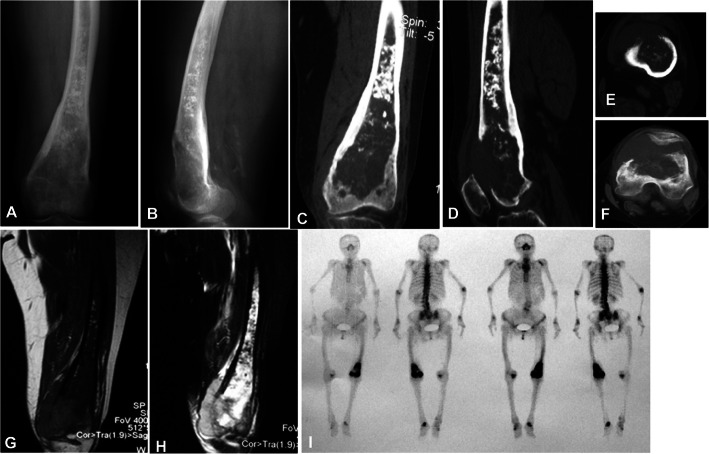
A 75-year-old woman had left leg pain for half a year and swelling of left knee for 5 months and was diagnosed to have dedifferentiated chondrosarcoma. a-f Plain radiograph (a and b) and computed tomography (c-f) displayed calcification within the medullary cavity, osteolytic destruction in the distal end, cortical destruction, and a soft tissue mass. g, h Magnetic resonance imaging in T1WI (g) and T2WI (h) showed unevenly long T1 and long T2 signal in the lesion. i. ECT scan demonstrated increased radionuclide uptake at the distal femoral segment and assembling of radionuclide at the distal femoral end with some areas of low uptake of radionuclide. This case showed the upper calcified chondroma and lower bone destruction with dedifferentiation
